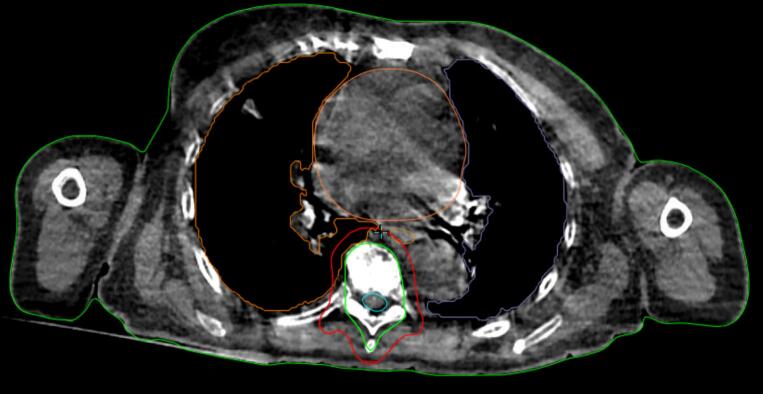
HyperSight cone-beam CT with AI-based organs at risk contouring and manual on couch clinical target volume (green) contouring used for treatment planning for patient 7. Planning target volume in red. (For interpretation of the references to colour in this figure legend, the reader is referred to the web version of this article.)
